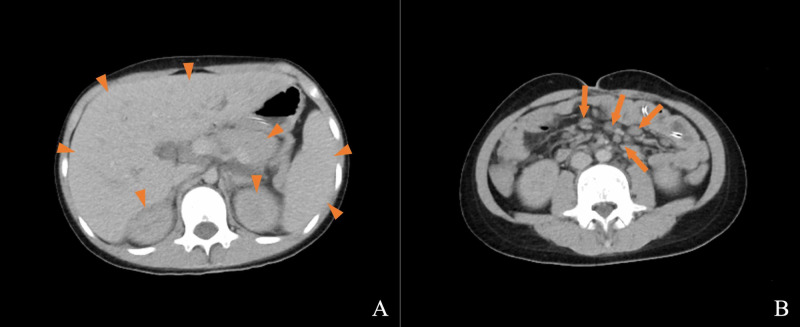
Contrast-enhanced abdominal CT image on the day of the onset of septic shock. Mild swelling was observed in the liver, kidneys, and pancreas (A, arrowhead), and multiple lymph nodes appeared enlarged (B, arrow). There were no obvious infectious findings such as abscesses or intestinal perforations.
